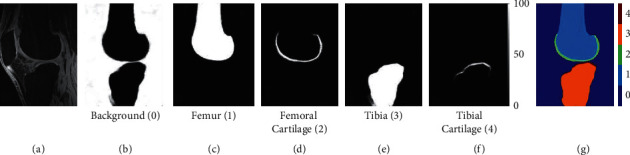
Example of knee bone and cartilage segmentation from pixelwise class probability with (a) original MR image, (b–f) background, femur, femoral cartilage, tibia, and tibial cartilage, with index 0 to 4, respectively, and (g) combination of each class index (adapted from [50]).
